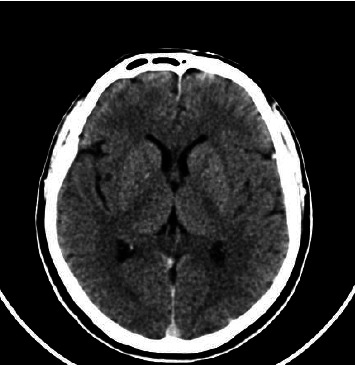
An axial noncontrast cranial CT image of the patient showing no abnormalities.
